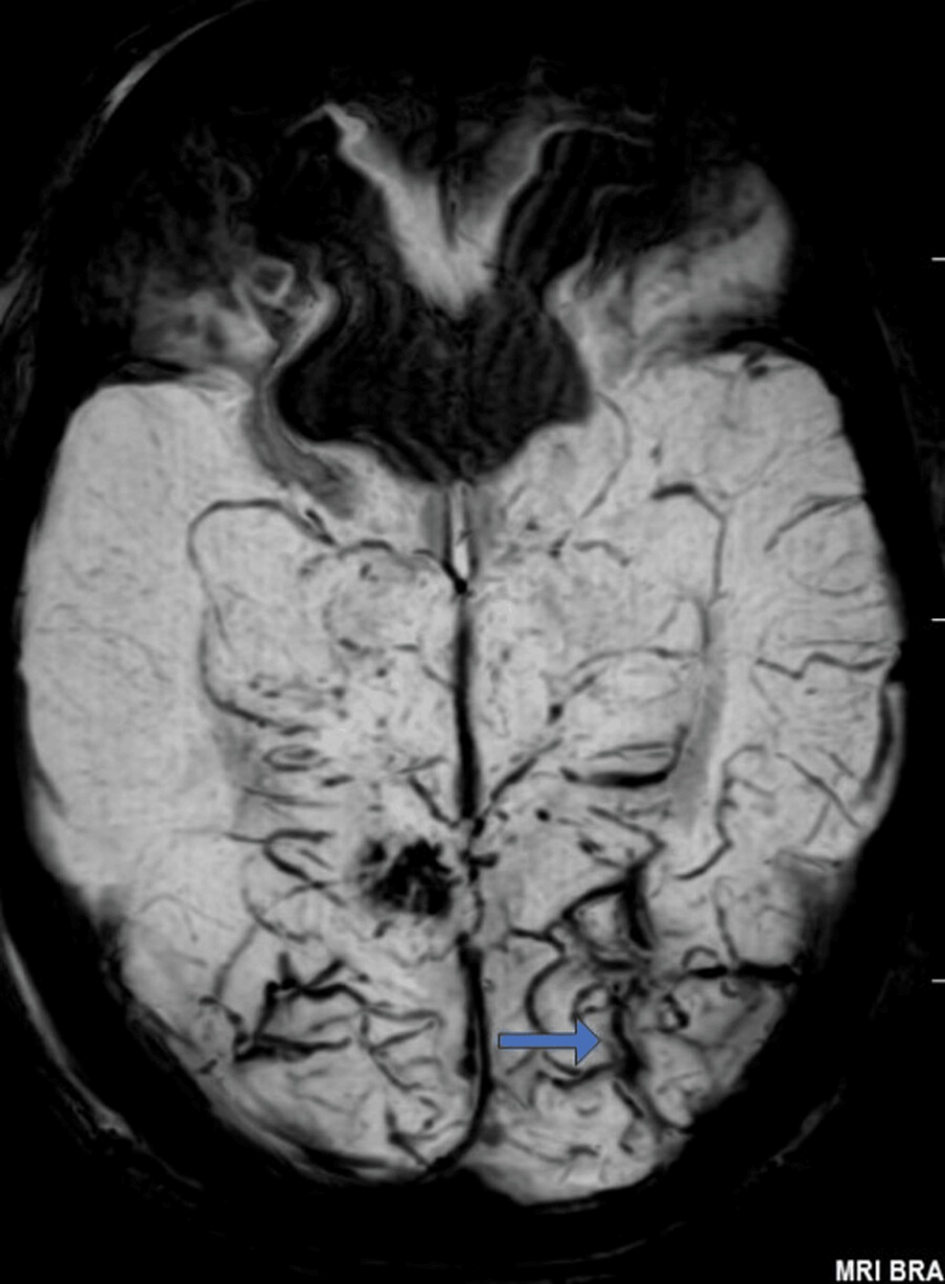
Magnetic resonance image of the brain of the patient in gradient echo sequence showing microhemorrhages in the posterior lobe of the brain (blue arrow).
